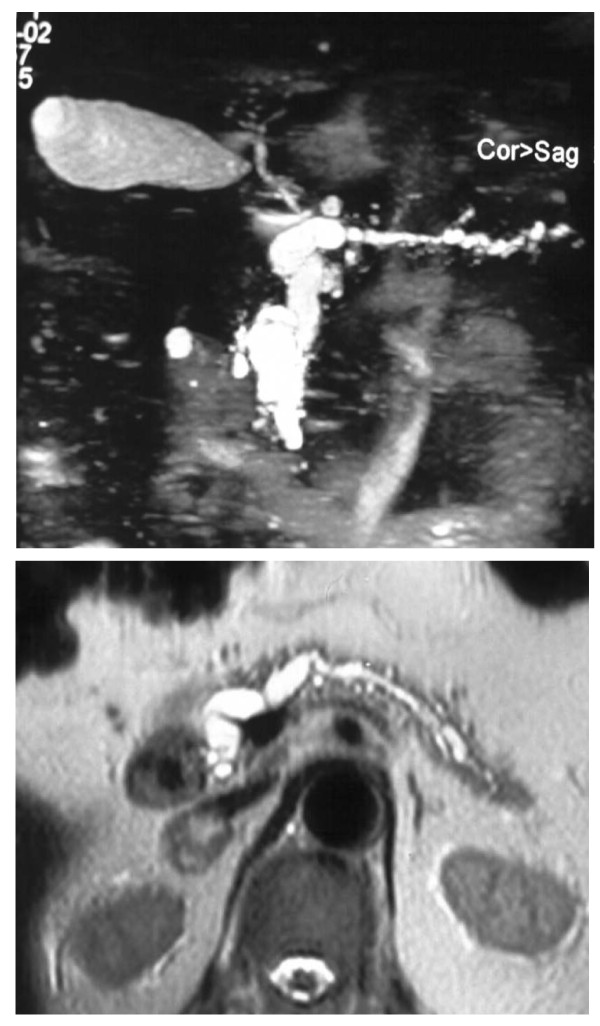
Colangio-MRI: main duct IPMN involving the whole gland, three-dimensional and axial reconstruction.
